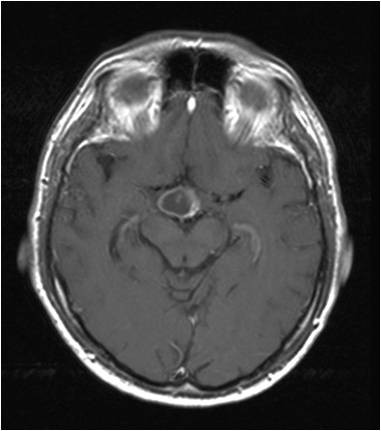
Axial brain MRI of a 51 year old male in a different slice.
